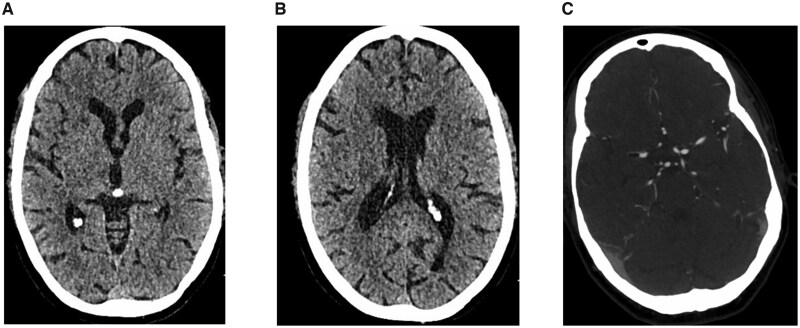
Initial imaging investigations. (A) CT head at ganglionic level showed a small focus of slightly decreased attenuation in the medial part of the right thalamus. (B) CT head at supraganglionic level revealed no obvious acute abnormalities. (C) CT angiogram showed a right M1 embolus with fat attenuation.
